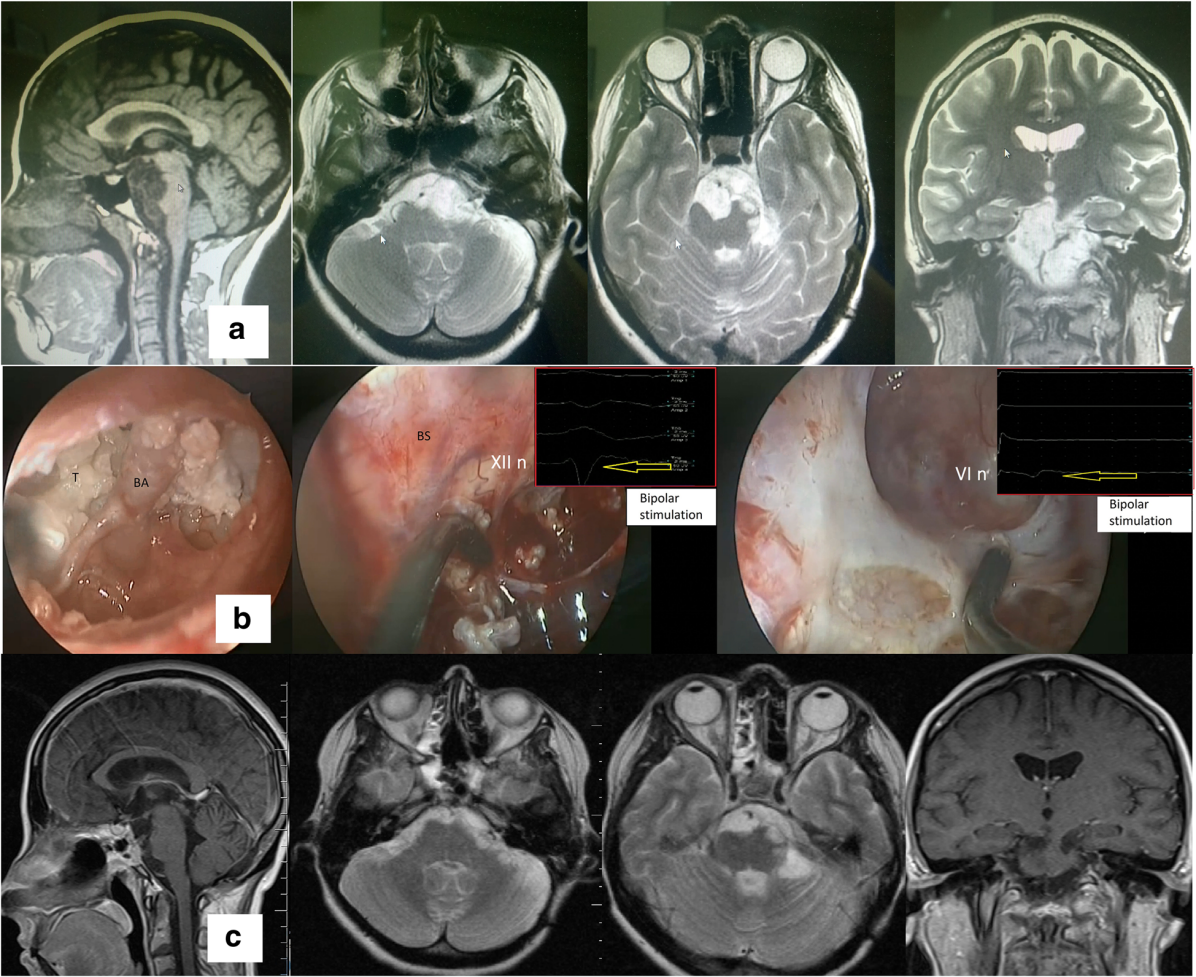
Clinical case. Patient no. 19 in Table 1. a MRI before surgery. Giant cholesteatoma in a 33 years old female patient (she was previously operated three times). b Intraoperative photograph. BA basilar artery, BS brain stem, T tumor. c MRI 4 months after surgery. Partial removal of the tumor
